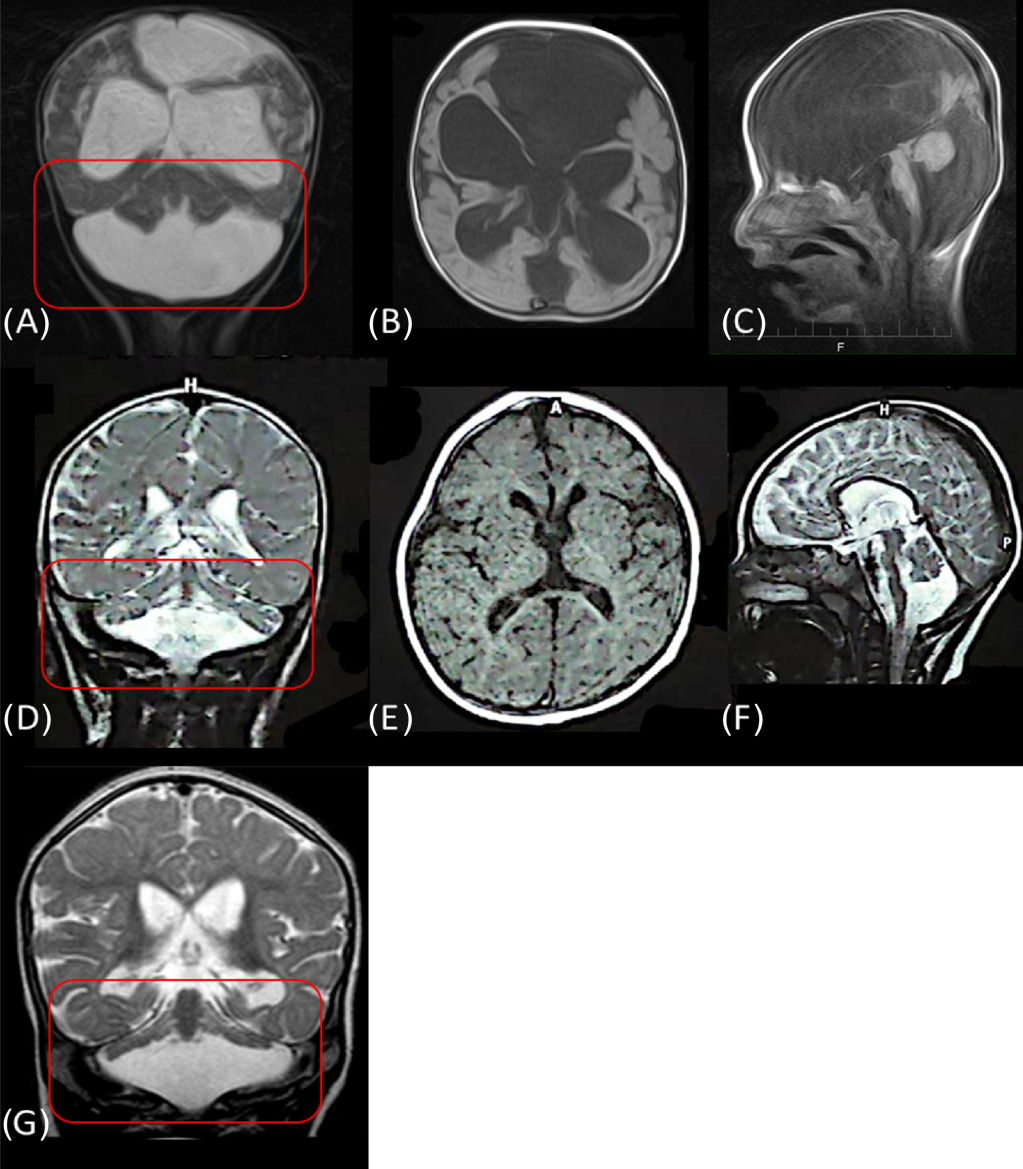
MRI findings of Family #201 compared with the literature of patients with PCH2A. A–C: MRI of L7274, carrying the class IV *MOCS2* variant (c.226G > A) in homozygous state showing a complex supra‐ and infratentorial brain malformation with enlarged cerebrospinal fluid space; D–F: MRI from patient L7270 with a confirmed pathogenic homozygous *TSEN54* variant (c.919G > T) with flattened cerebellar hemispheres (circled red), also known as dragon sign. G: dragon sign of a patient with the same homozygous variant as patient L7270 [8].
